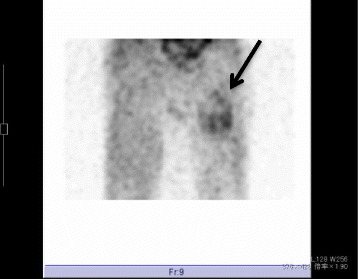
Thallium-201 scintigraphy showing uptake at the area of tumor (see *arrow*)
